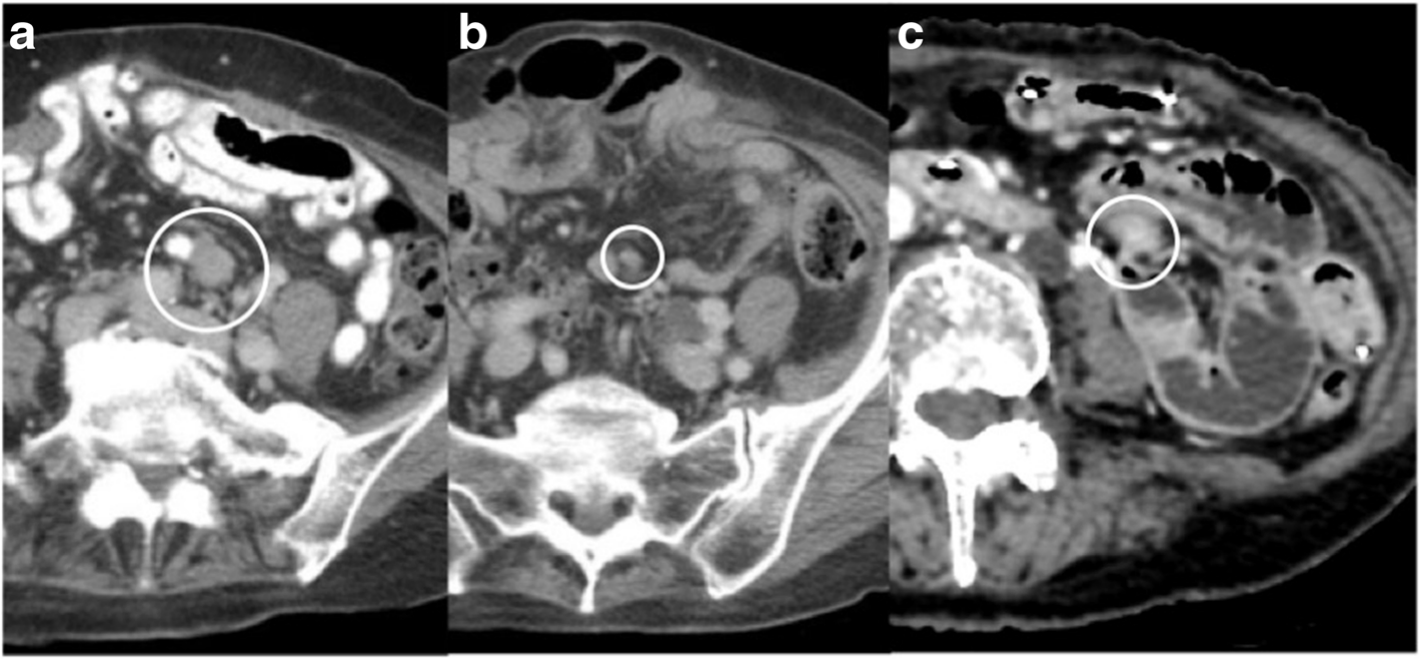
Case 1 imaging findings. a Abdominal CT at study enrollment. b Abdominal CT 3 months after start of nilotinib therapy. c Abdominal CT of the developing nilotinib-resistant tumor
